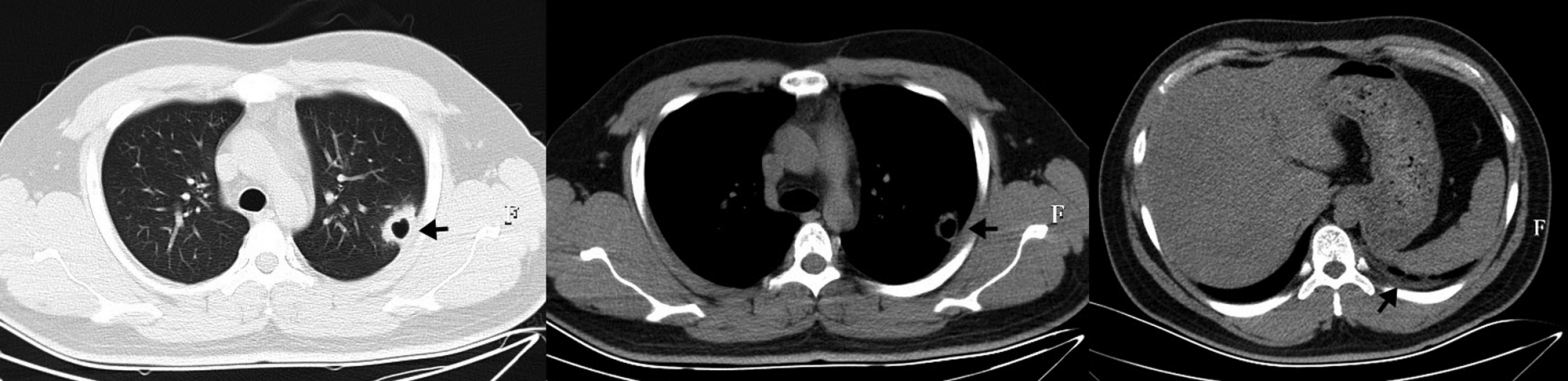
Chest CT before treatment, showing a thick-walled cavity in the left upper lung, with smooth inner and irregular outer margins. The adjacent pleural and the left lower pleural were thickened.
